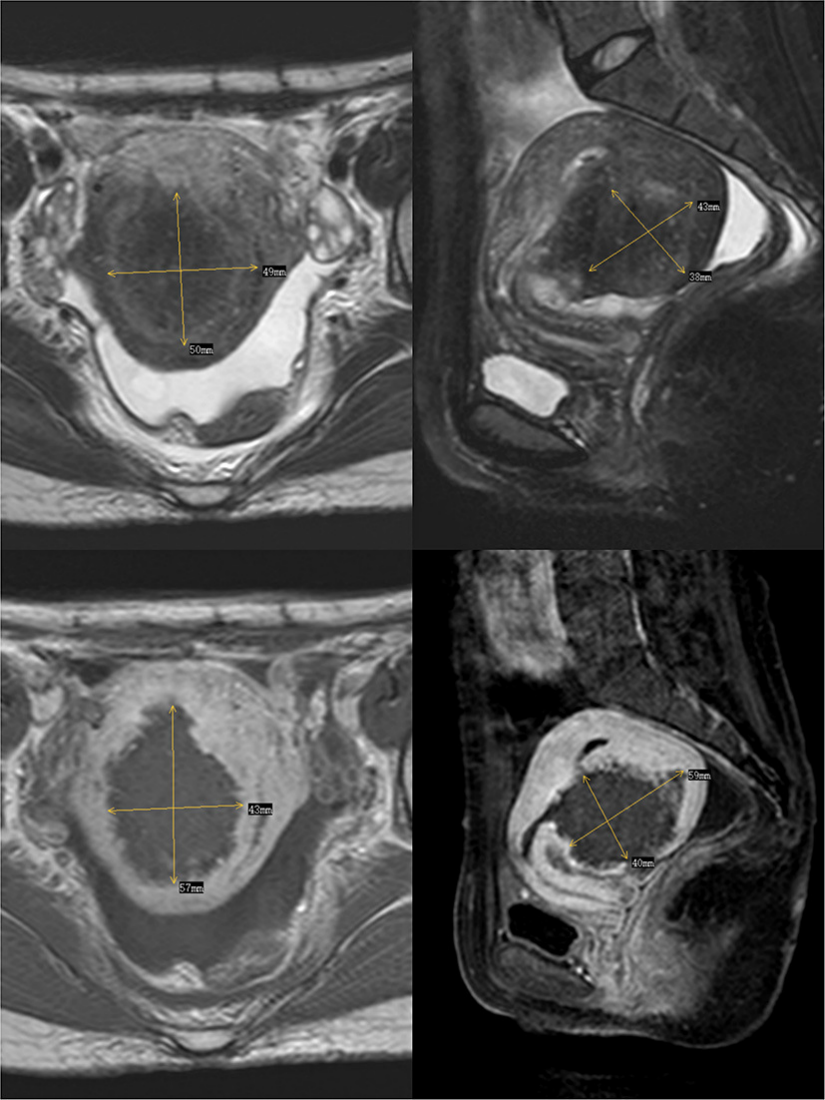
The above two images are T2-weighted images after the ablation of Haifu, while the following two images are enhanced images of T1. The filling defect is where part of the lesions were ablated by Haifu.
